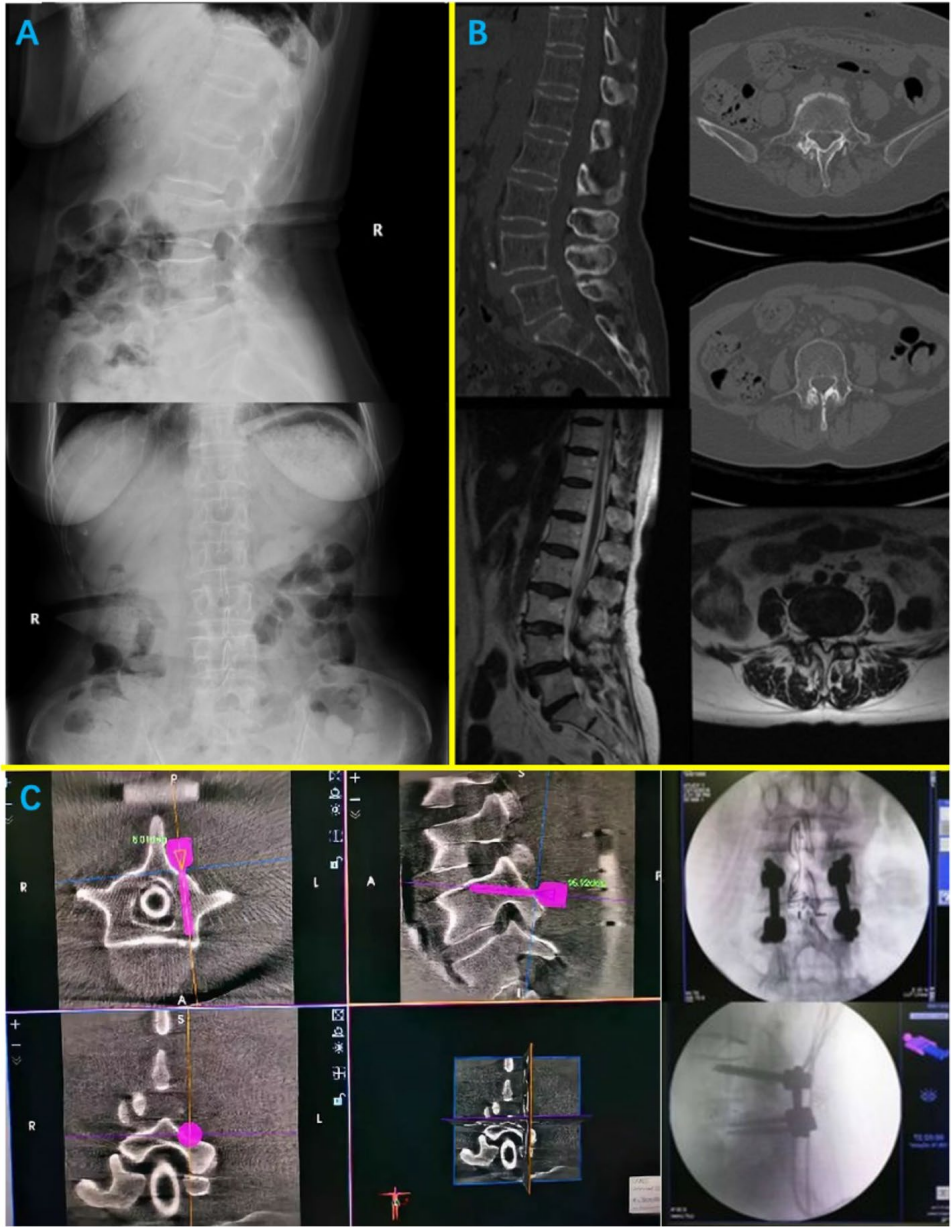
A Preoperative X-ray of patient; B Preoperative CT showed L4/5 spondylolisthesis facet joints hyperplasia and degeneration, MRI showed L4/5 spinal stenosis. C Robot-assisted intraoperative positioning and intraoperative fluoroscopy
